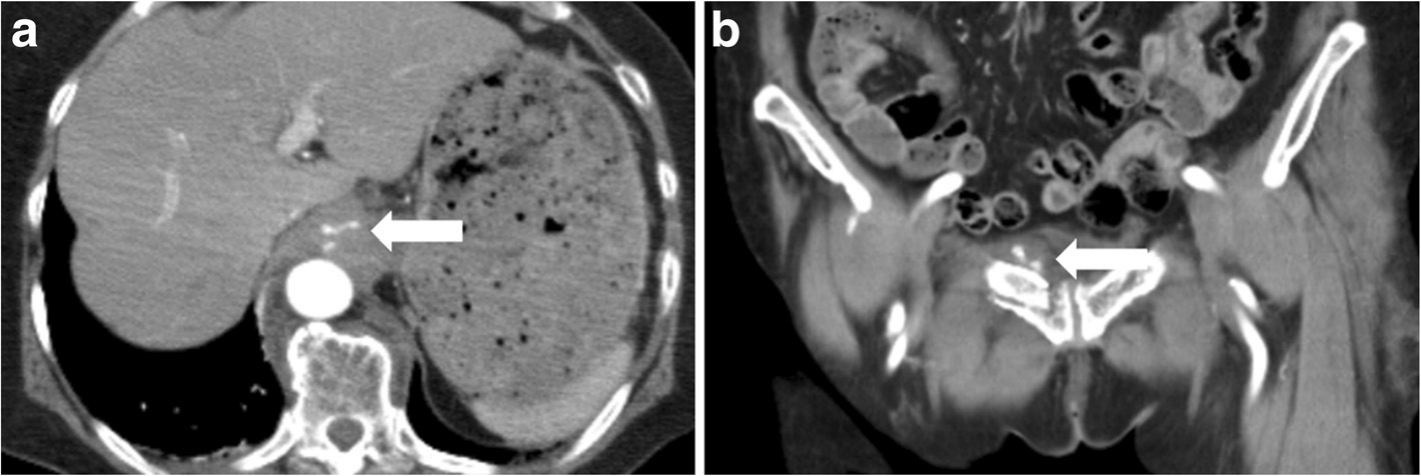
a Enhanced CT revealed contrast material extravasation within the retrocrural space on arterial phase (arrow). b Enhanced CT revealed contrast material extravasation above the pubic bone fractures on arterial phase (arrow)
